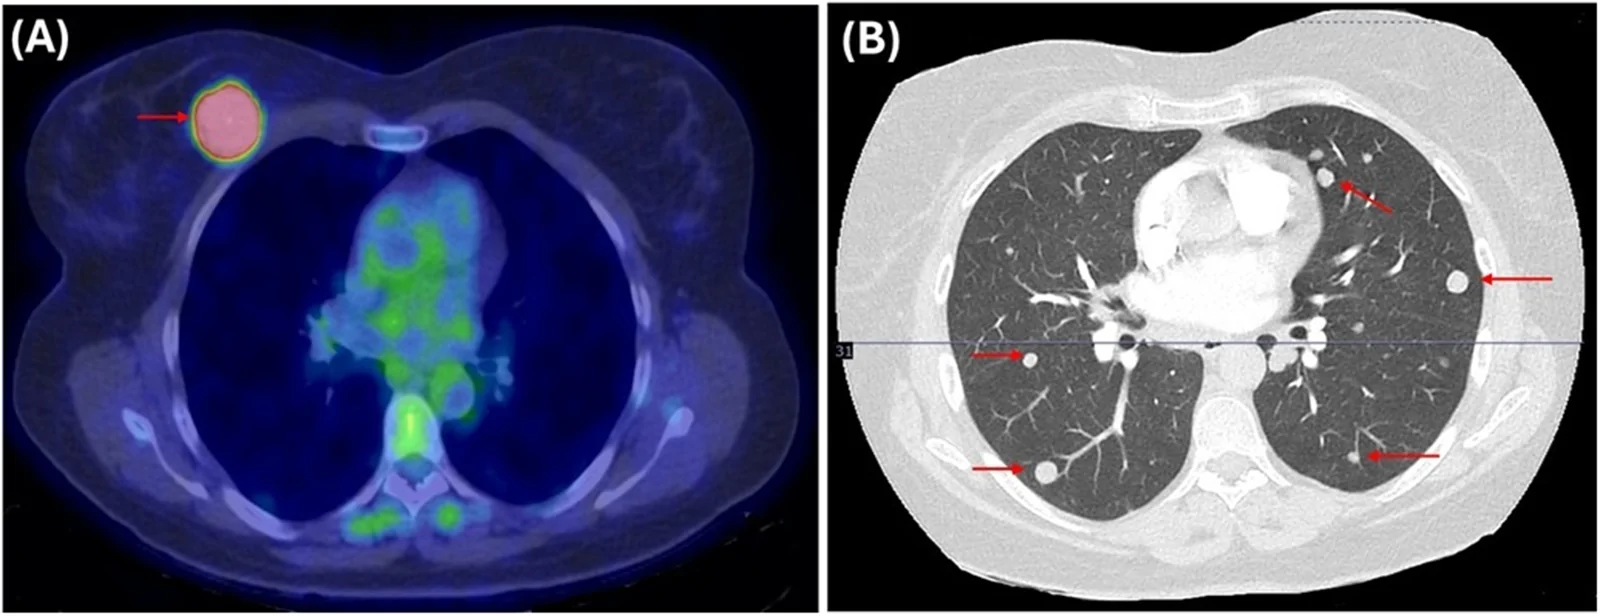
(A) Primary tumor located in a breast of TNBC patient #1 detected by positron emission tomography/ computed tomography (PET/CT) imaging scan performed with the radiotracer [F18]-fludeoxyglucose (FDG) before any treatment. (B) Lung metastases were detected six months after RT by CT angiogram imaging. Red arrows indicate the location of primary tumor and metastases.
